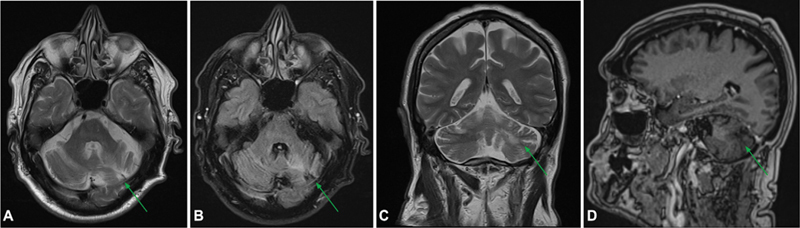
Images after the treatment with vemurafenib showing an area of sequelae in the left cerebellar hemisphere, characterized by volumetric reduction and T2/FLAIR hyperintensity (
